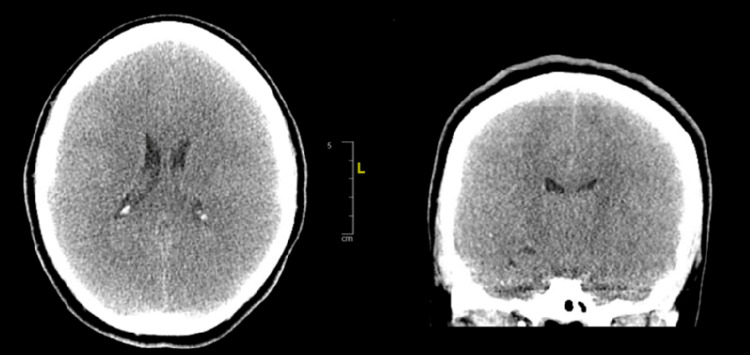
CT brain axial (right) and coronal (left) sections
